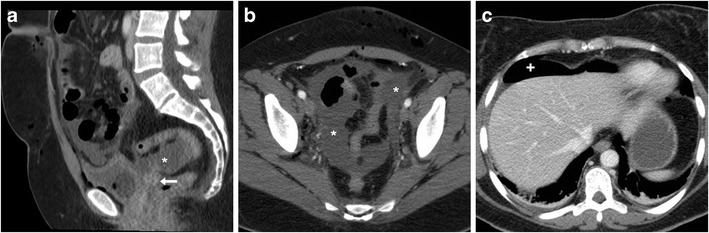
Peritonitis developing 4 days after LH. Contrast-enhanced CT images showed normal findings at the vaginal dome (arrow in A), moderate pelvic effusion (*) with thin serosal enhancement, and residual intraperitoneal air (+). Laparotomy identified a focal ileal perforation which was repaired
